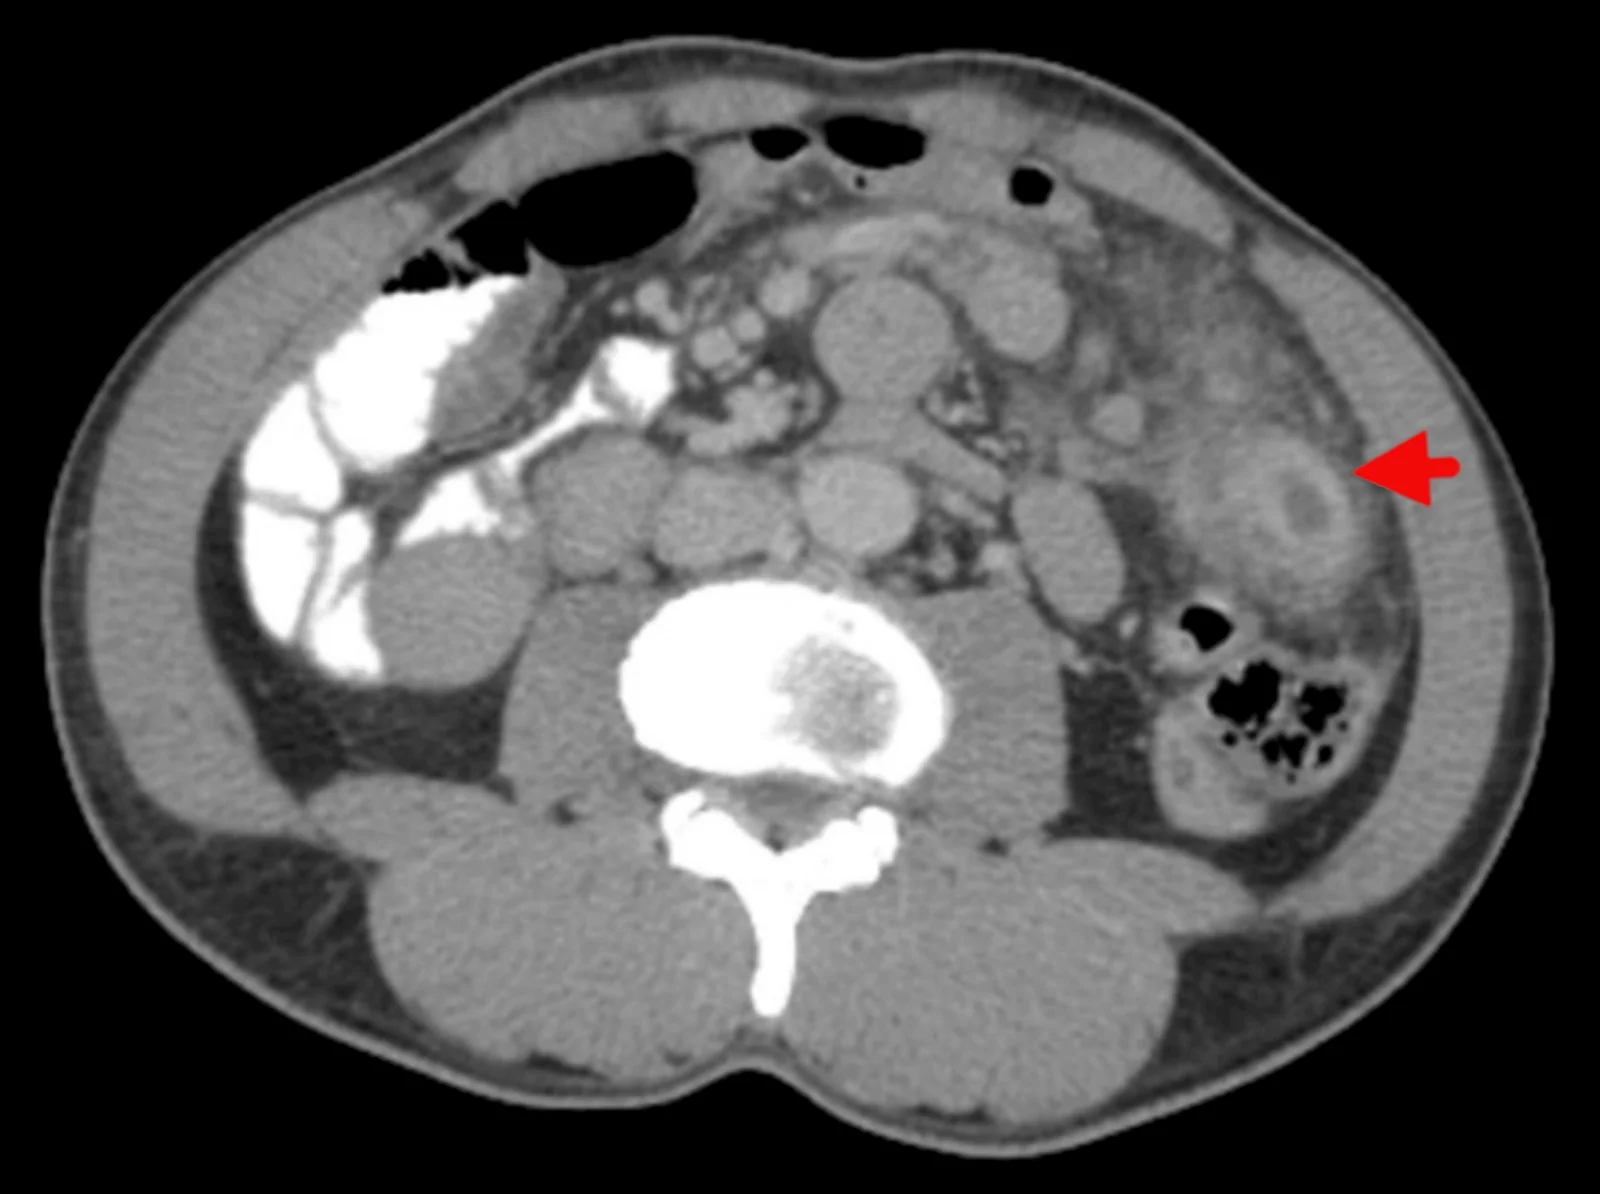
Acute appendicitis on axial CT Axial contrast-enhanced CT image demonstrating a left-sided dilated fluid-filled appendix, with surrounding inflammatory fat stranding, consistent with left-sided acute appendicitis.
